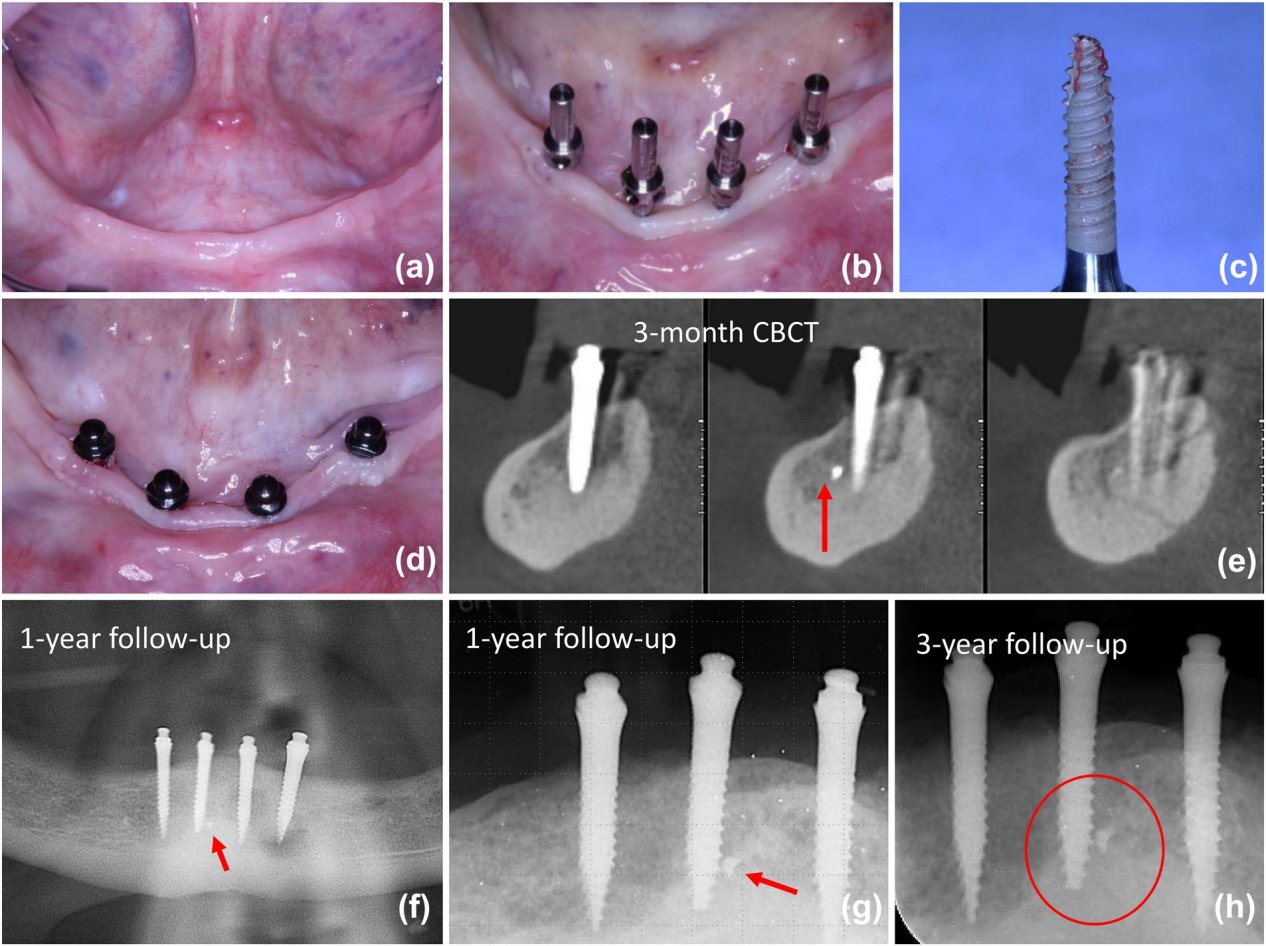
(a) Pre surgery mandibular view; (b) Flapless transoperative view after bone preparation and the paralleling posts in position; (c) Mini implant with apical fracture; (d) Immediate post‐surgery view; (e) CBCT image scans at the 3‐month follow up showing a satisfactory osteointegration and the fractured fragment located in a dense cortical bone region; (f) Panoramic radiograph at the 1‐year follow‐up; (g) Periapical radiograph of the central right implant at the 1‐year follow up, evidencing the osseointegration of the fractured fragment (h) Periapical radiograph at the 3‐year follow up, with no signs of bone alterations.
